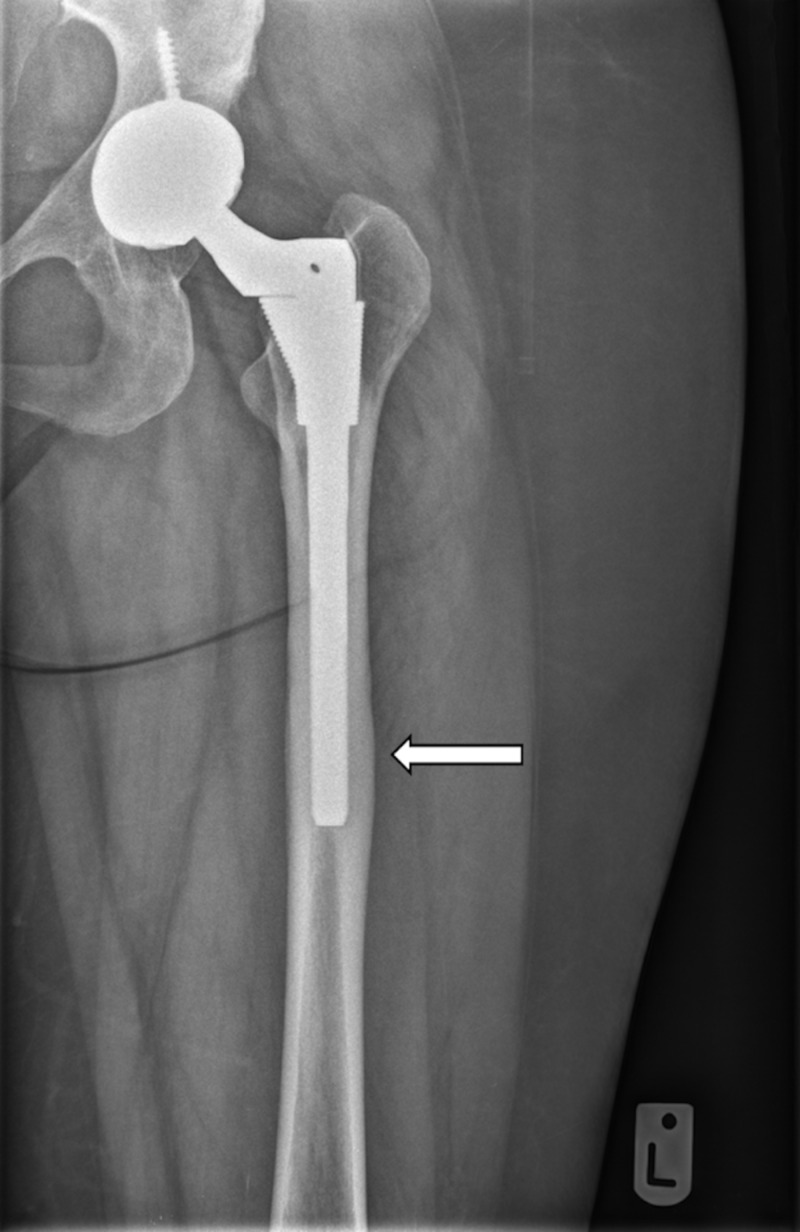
AP radiograph of left hip, S-ROM femoral stem and Pinnacle acetabular cup, with cortical hypertrophy in zone 3 (white arrow). AP, anteroposterior
